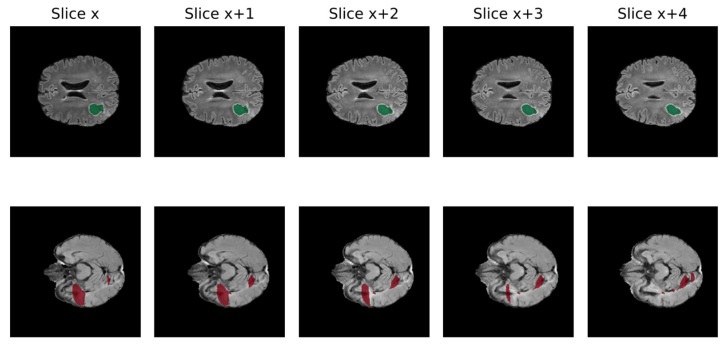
Qualitative comparison of processed FLAIR from volumetric raw input (above) and non-volumetric one in 5 different slices of the same MRI (Green: cavity, Red: FLAIR hyperintensity) [46].
